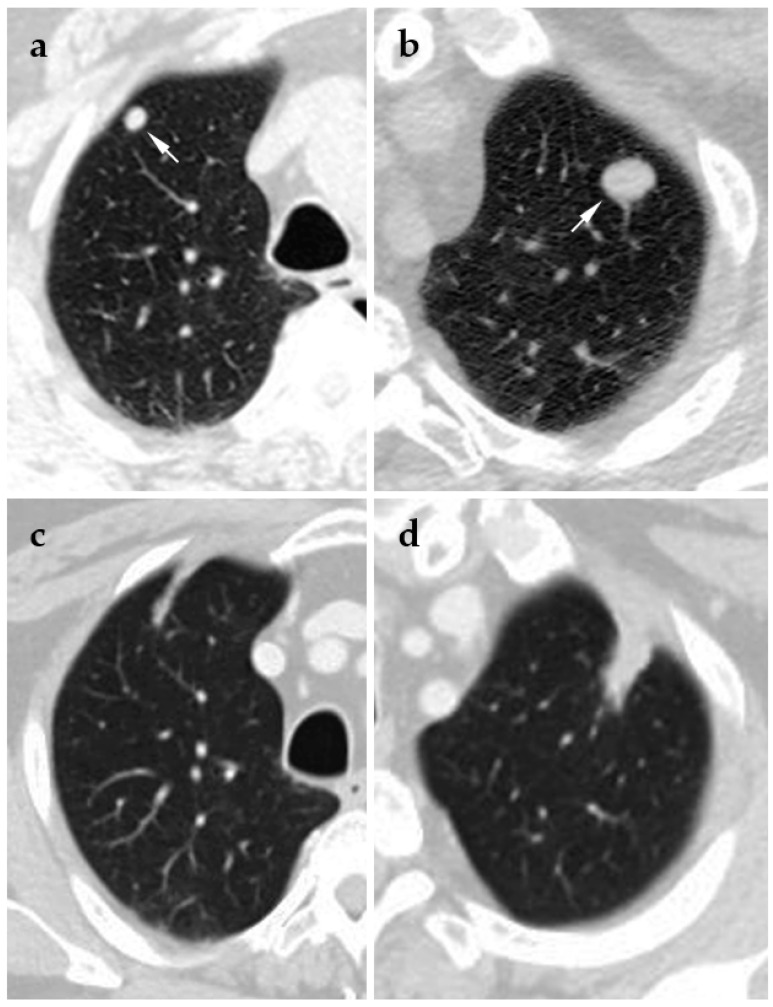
65-year-old male presents with a history of end-stage liver disease secondary to chronic hepatitis C complicated by hepatocellular carcinoma. Patient underwent an orthotopic liver transplantation. Four years after the transplant, the patient underwent a biopsy of a lung lesion, which was positive for metastatic hepatocellular carcinoma. The patient then underwent cryoablation of the left lung, followed by cryoablation of the right lung one month following initial ablation. When treating bilateral lung lesions, the authors ablate one lung at a time to avoid the risk of bilateral pneumothorax. (a) Supine axial pre-ablation CT of the right upper lobe nodule (white arrow indicates the lesion to be ablated); (b) supine axial pre-ablation CT of the left upper lobe nodule (white arrow indicates the lesion to be ablated); (c) supine axial follow-up CT of the right upper lobe at 18 months post-ablation; (d) supine axial follow-up CT of the left upper lobe at 18 months post-ablation.
